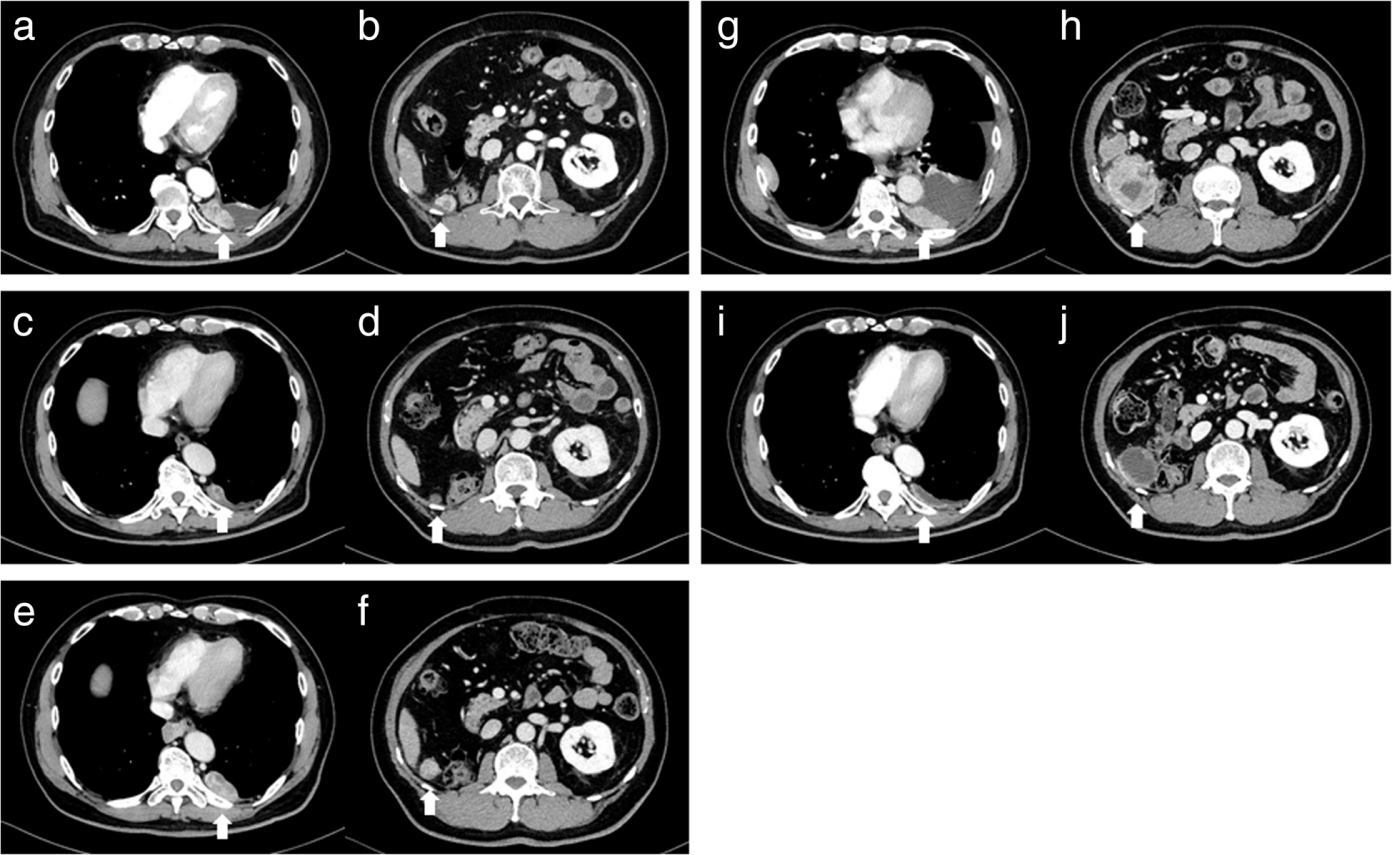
Computed tomography findings during therapeutic courses. a, b New metastases such as pleura, diaphragm, and the right paracolic gutter appeared after sunitinib treatment. c, d The metastases diminished in size and lung metastases maintained shrinking state after axitinib treatment. e, f The size of metastases increased again after discontinuation of axitinib. g, h Existing metastatic lesions were growing, peritoneal dissemination appeared in the pelvic region, and pleural effusion appeared after nivolumab treatment. i, j Metastases such as lung, pleura, diaphragm, and the right paracolic gutter diminished in size after low-dose axitinib (2 mg/day) treatment
